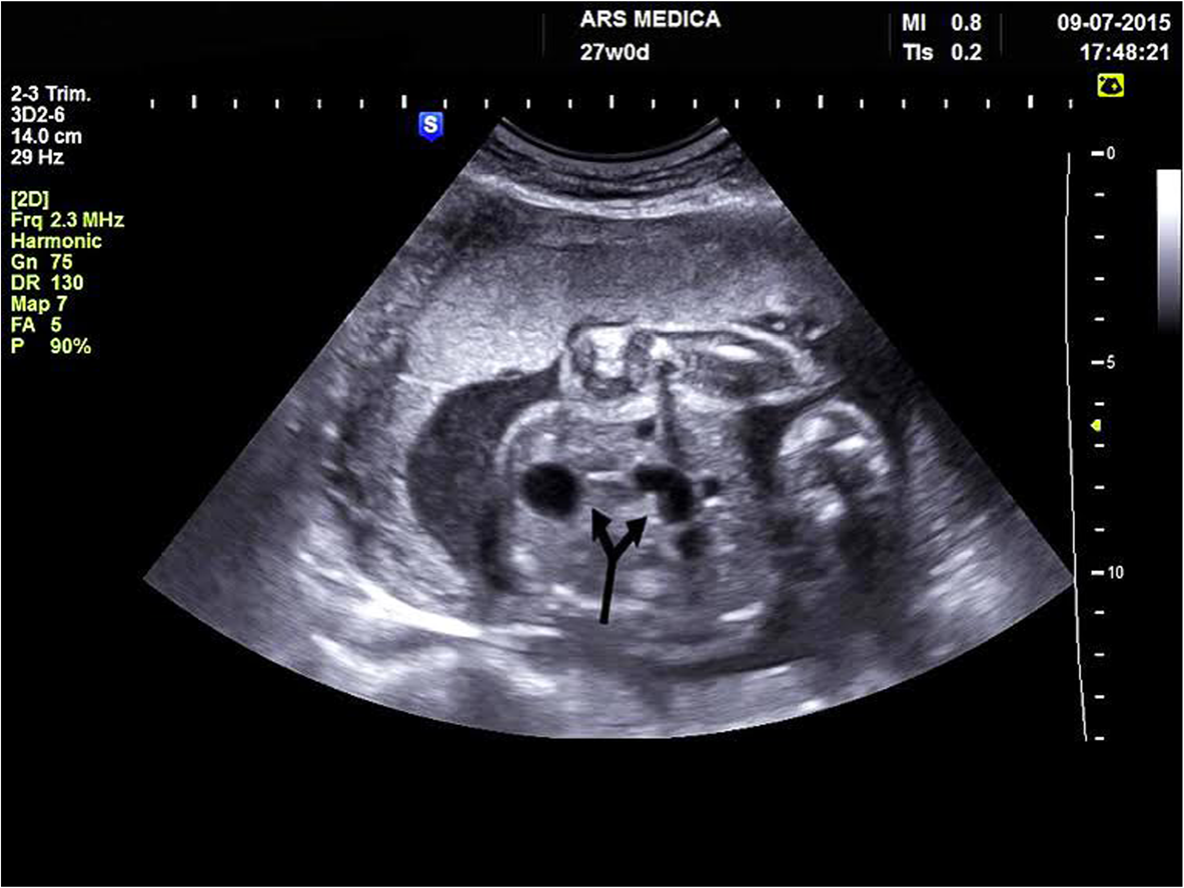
Antenatal ultrasonography 27 th week GA revealed several hypoechoic formations corresponding to dilated stomach and small bowel
